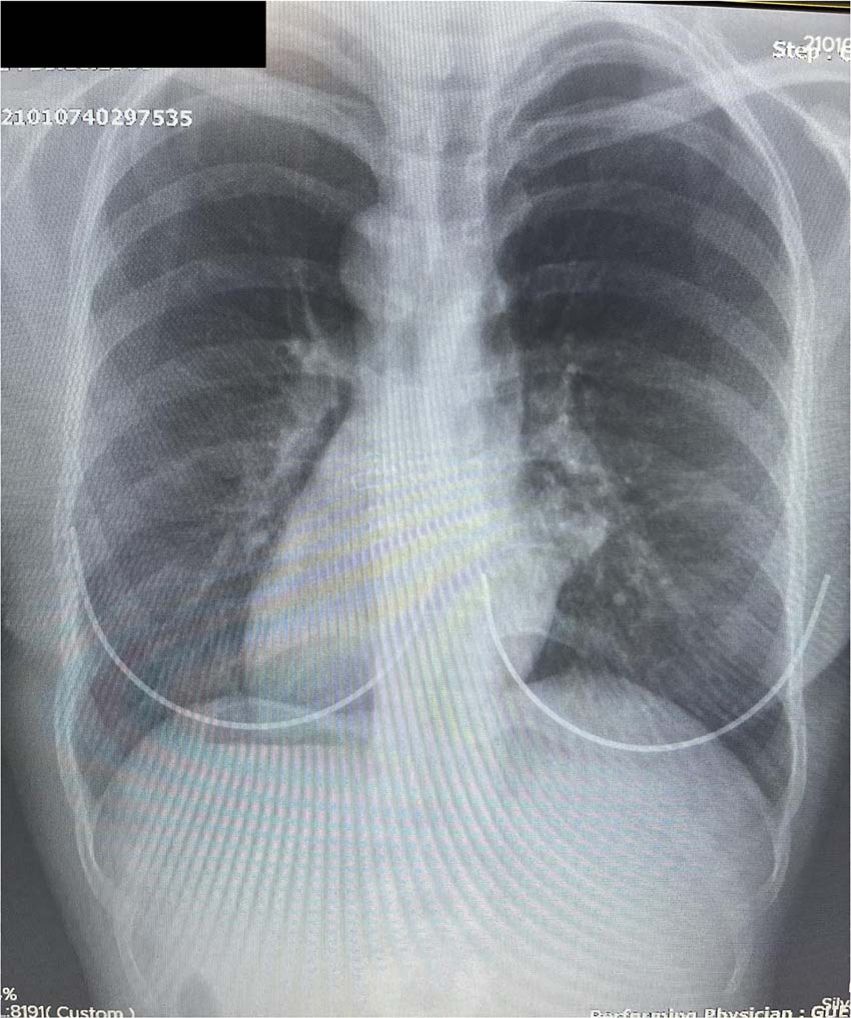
Chest Radiograph (Posteroanterior View): Chest X-ray showing dextrocardia with a right-sided cardiac apex and normal positioning of the mediastinum toward the right. The gastric bubble is visible on the right, supporting the diagnosis of situs inversus totalis.
